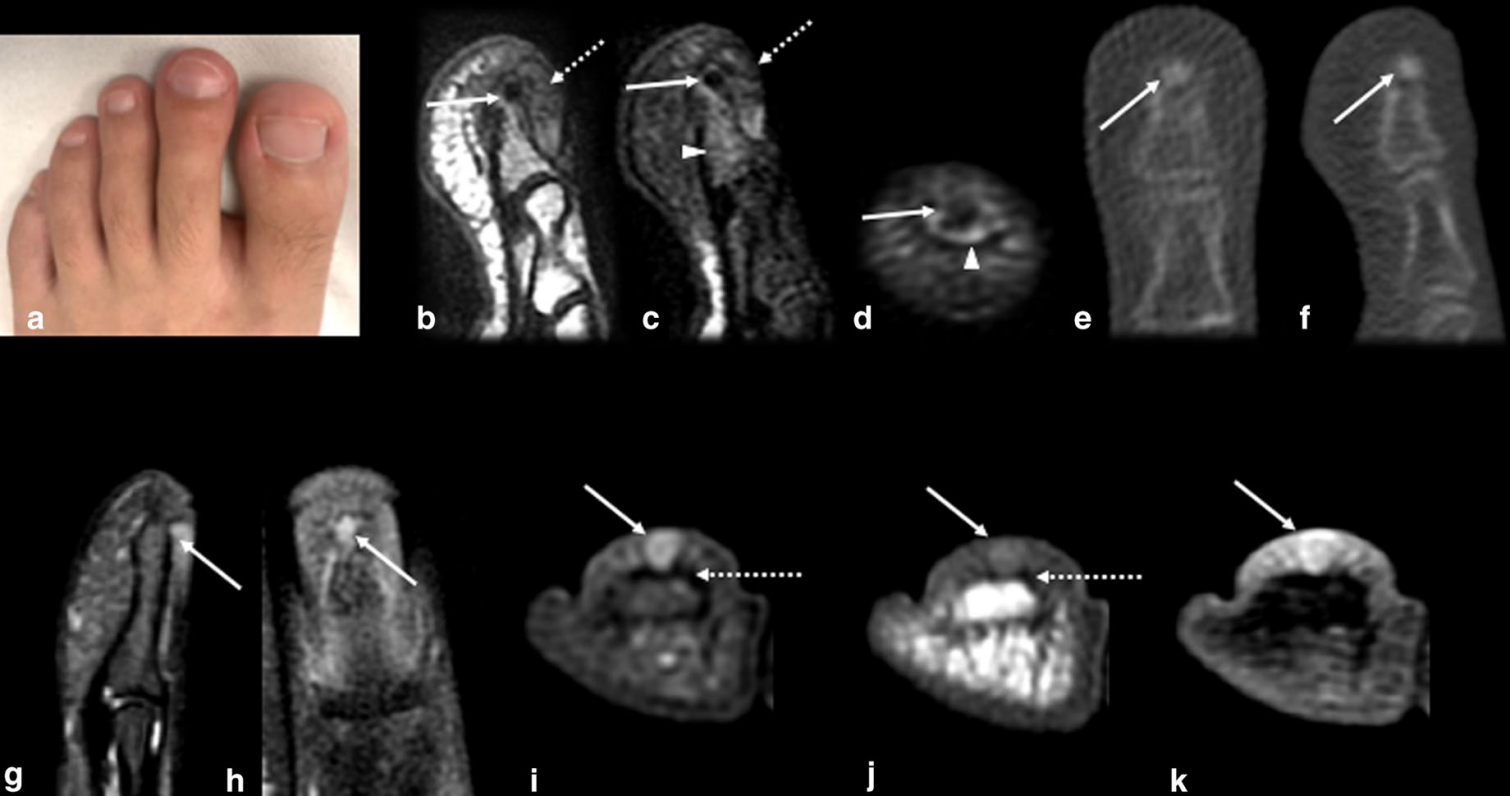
Osteoid osteoma versus glomus tumor. (a–f) Male, 19 years old, presenting with second toe clubbing and night pain (a). Sagittal T1 (b) and sagittal (c) and axial (d) T2FS MR images showed diffuse nail bed thickening (dotted arrows), with no defined nodule, as well as a low signal cortical/juxtacortical nodule at the distal phalanx (arrows), accompanied by bone marrow edema (arrowhead). A CT scan (e, f) was performed, and the findings revealed a sclerotic nodule corresponding to an OO nidus (arrows), with minimal reactional surrounding sclerosis. (g–k) Female, 53 years old, presenting with pain on the 4th finger that radiated to the forearm. Sagittal, coronal, axial T2 FS, axial T1 and T1 FS GD MR images depicted a subungual nodular well-defined lesion (arrow) with remodeling of the subjacent phalanx cortex (dotted arrow) and homogeneous enhancement after gadolinium injection (k). Note that diffuse thickening of the nail bed or phalanx sclerosis was absent.
